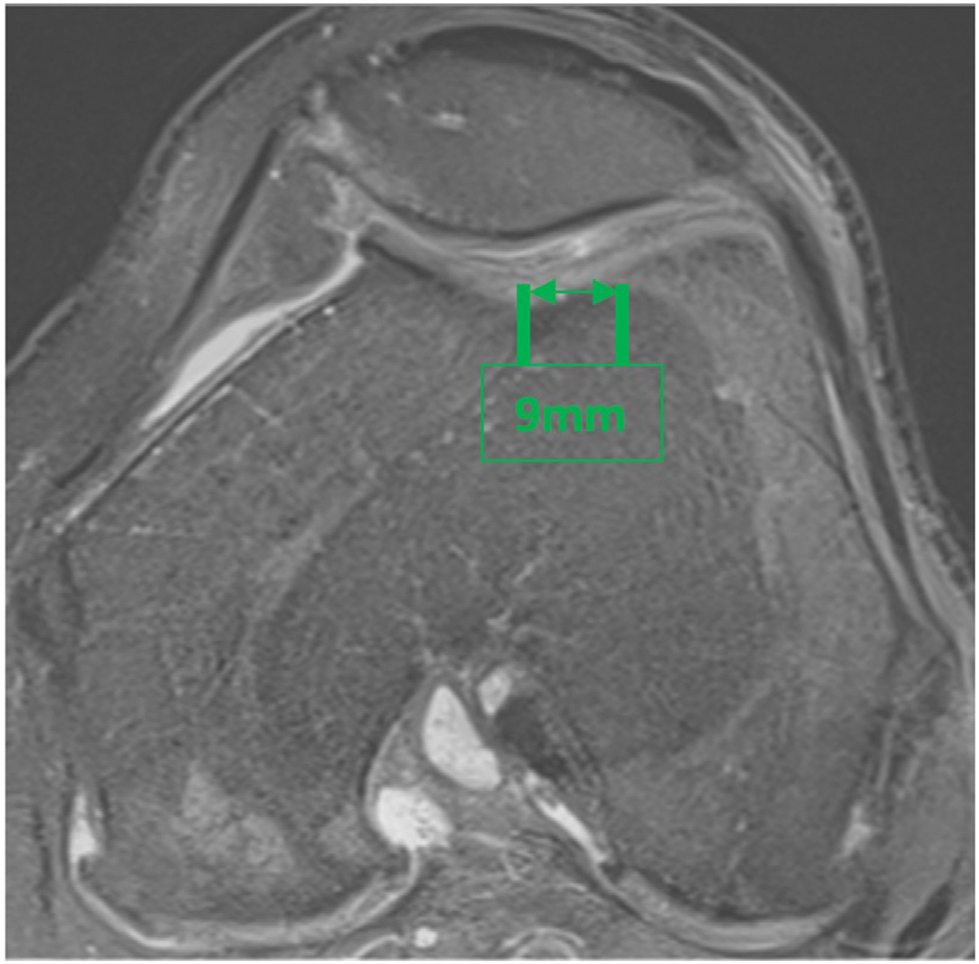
A preoperative MRI demonstrated intact patellofemoral cartilage. The tibial tubercle-trochlear groove distance was 9 mm. MRI, magnetic resonance imaging.
